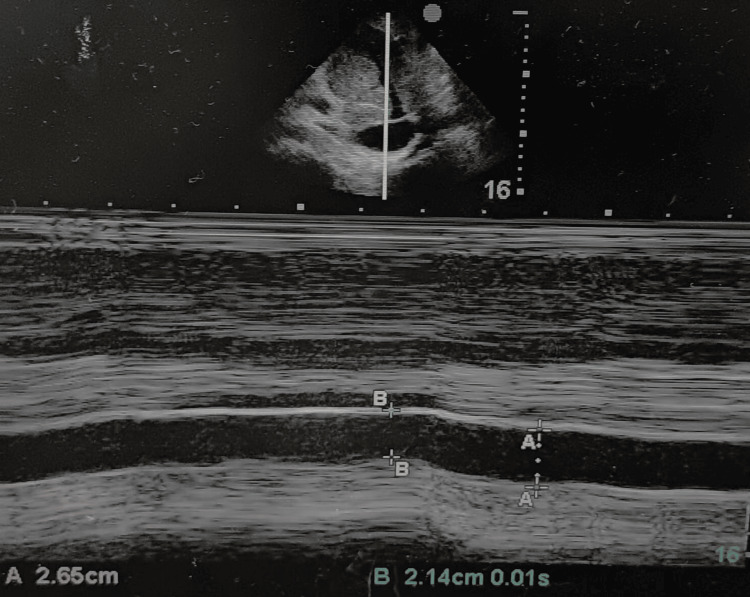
Point-of-care ultrasound showing a dilated inferior vena cava of 2.65 cm diameter with <50% collapsibility
